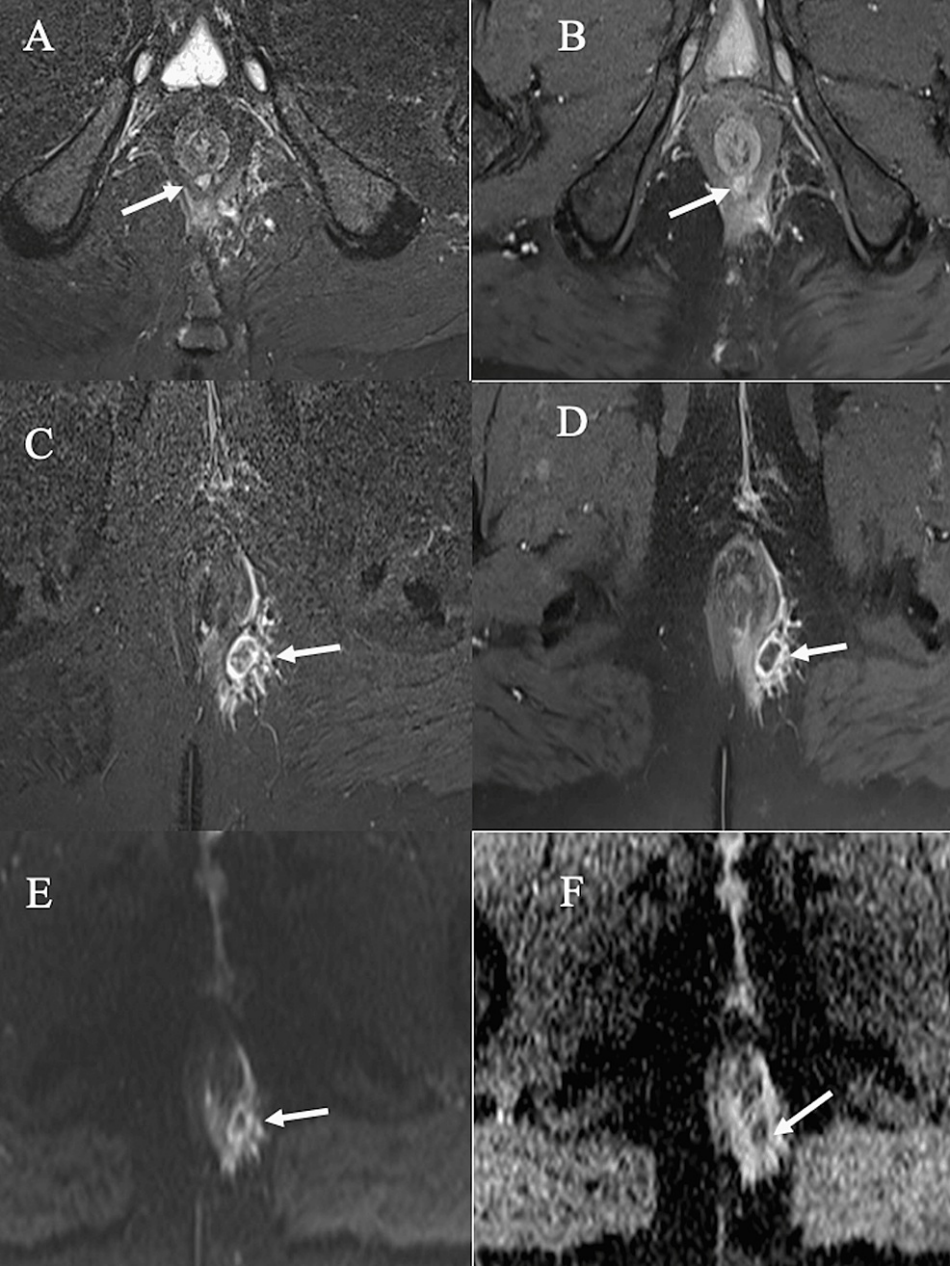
Grade 4 trans-sphincteric fistula with an abscess. (A-B) Axial STIR and post-contrast fat-suppressed T1-WI, respectively, showing grade 4 trans-sphincteric fistula crossing the external sphincter at the 5 o’clock position. (C-F) Axial STIR, post-contrast fat-suppressed T1, DWI, and ADC map, respectively, depicting abscess showing high signal intensity on STIR, peripheral rim enhancement on post-contrast fat-suppressed T1-WI and restricted diffusion in DWI and ADC map. DWI: diffusion-weighted imaging, STIR: short tau inversion recovery; ADC: apparent diffusion coefficient
